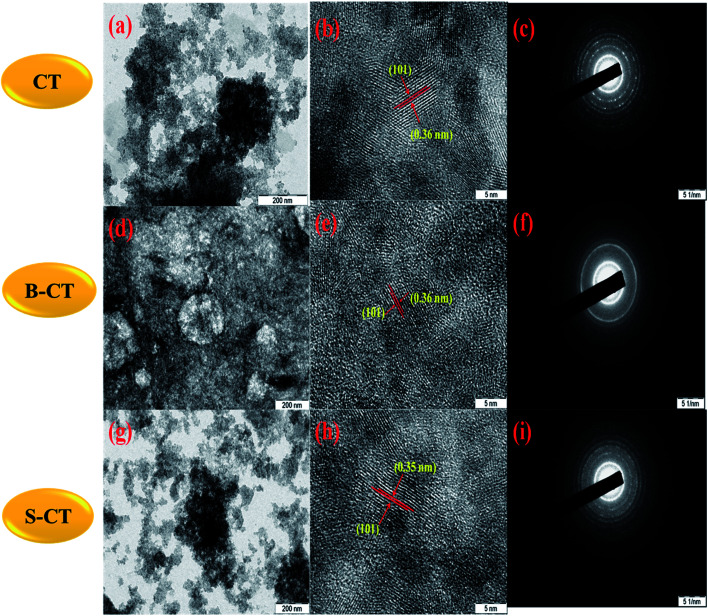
TEM, HRTEM and SAED images of ((a), (b) and (c)) CT, ((d), (e) and (f)) B-CT and ((g), (h) and (i)) S-CT.
